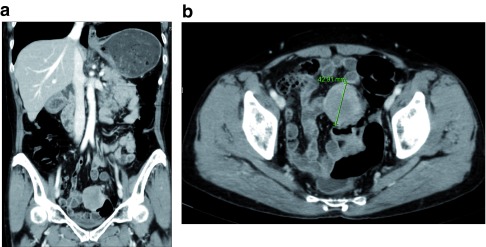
Abdominopelvic computerized tomography (CT). A well-circumscribed, well-enhanced, round-shaped mass was identified at the sigmoid colon. (
